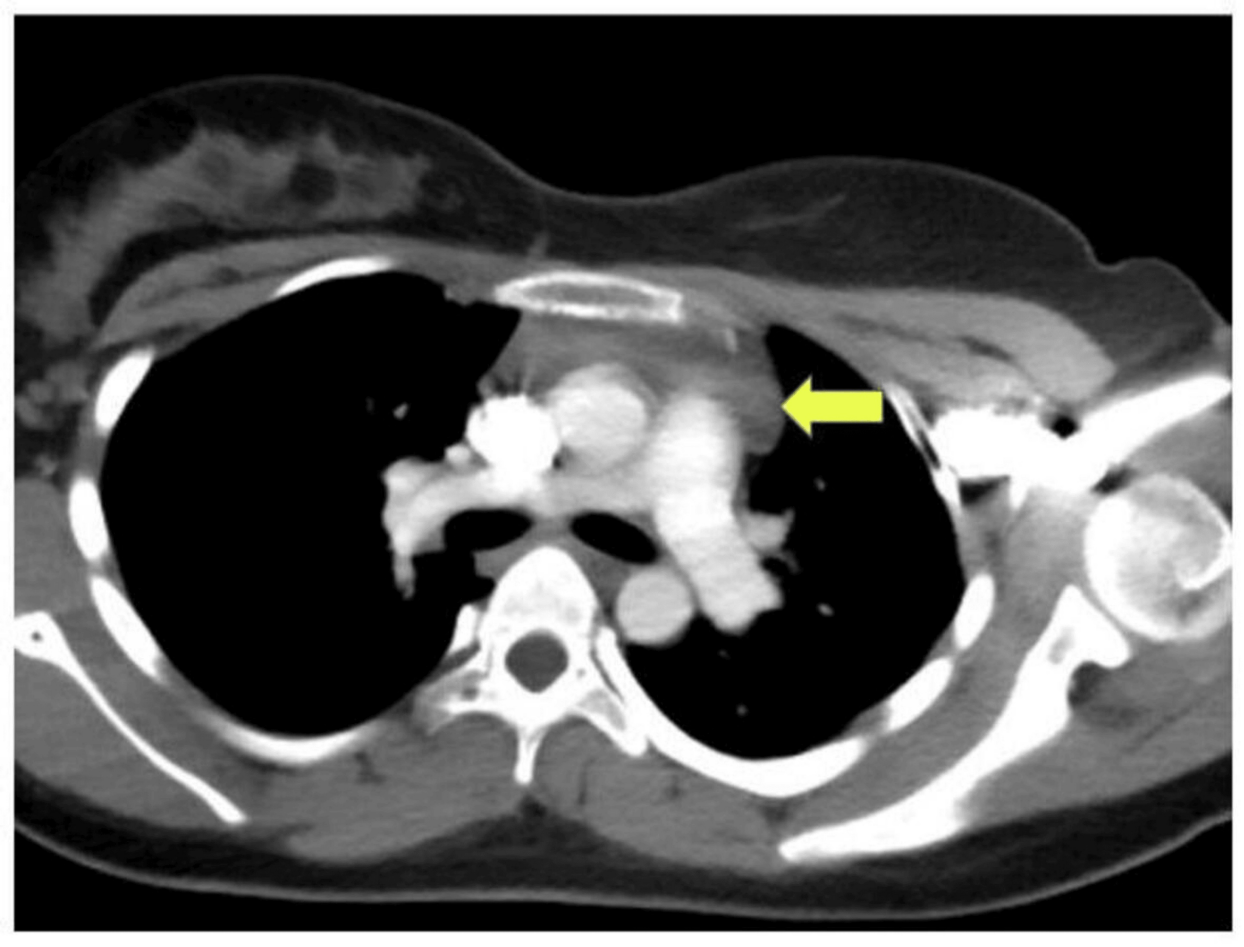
CT chest with contrast showing a bilobed mass with fat density and outer convex margins noted in the anterior mediastenum - thymic hyperplasia
